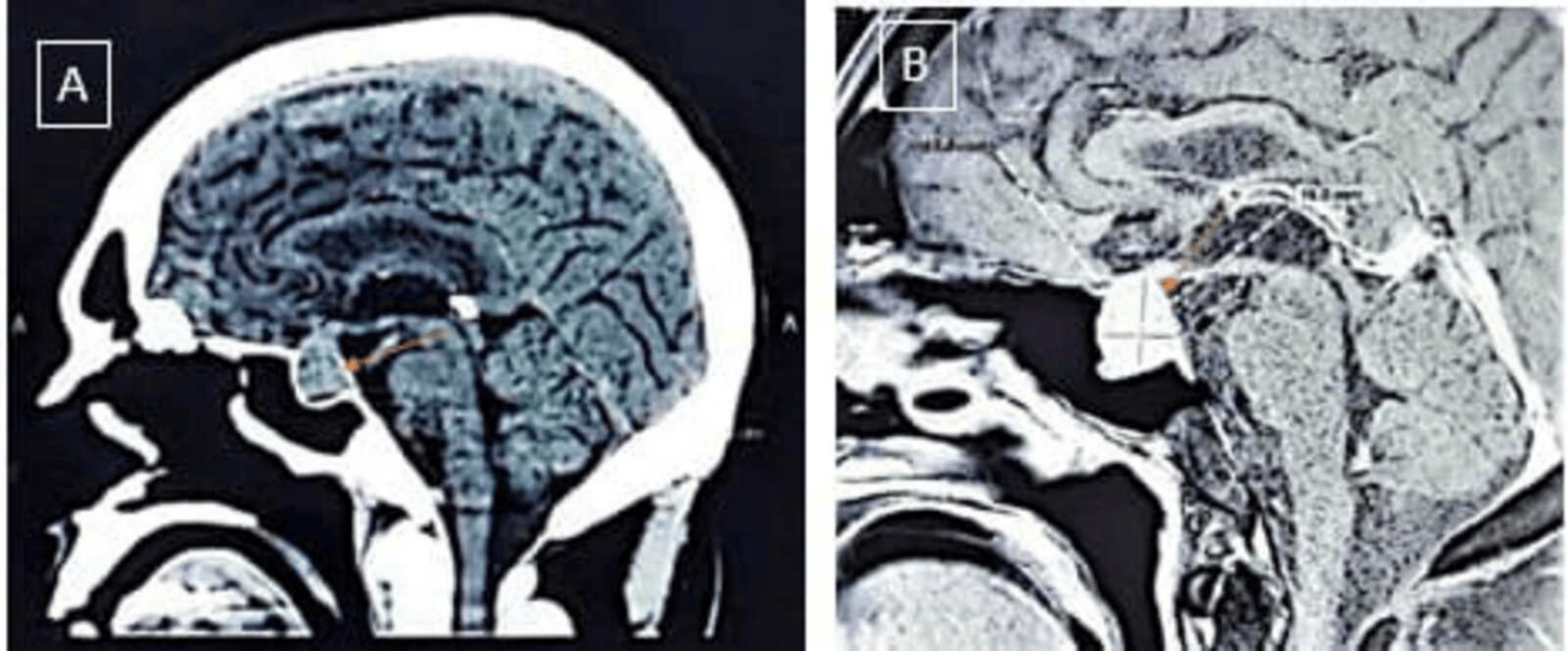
Sagittal view of imaging studies depicting a sellar mass. Panel A displays a CT image, while panel B shows an MRI image. Both images highlight the mass’s extension into the suprasellar region and sphenoid sinus.
